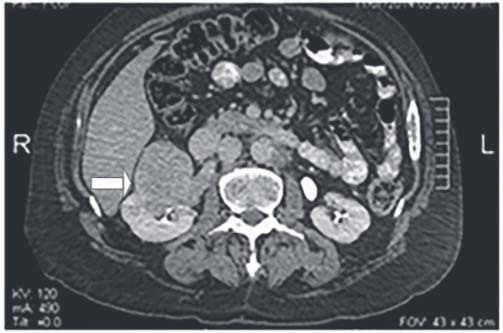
CT, where a solid formation of 53 x 56 mm is observed in the right kidney with microcalcifications. Cortical atrophy and a decrease in its size, with ureteropelvic dilatation of 20 mm.
